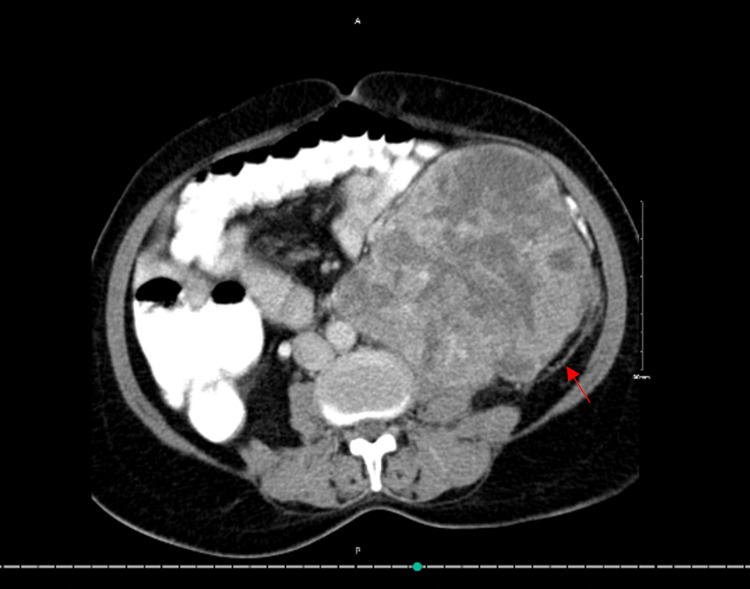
CT scan (axial view). The red arrow shows Leiomyosarcoma invading the nearby structures
